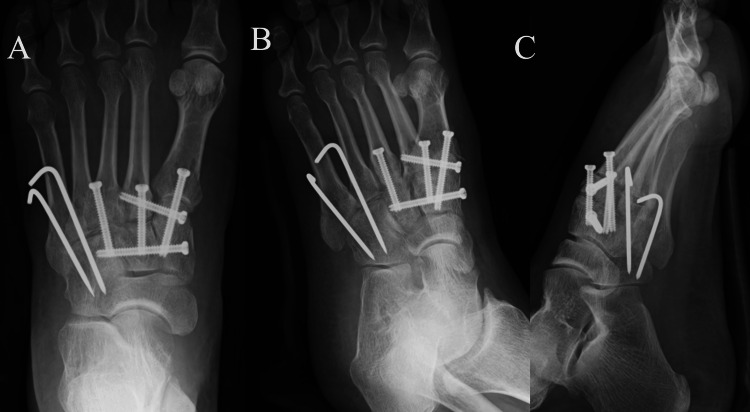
Postoperative radiographs demonstrating definitive fixation (A) Anteroposterior view. (B) Medial oblique view. (C) Lateral view. Cannulated cancellous screws and Kirschner wires (K-wires) were used to stabilize the Lisfranc joint and maintain anatomical alignment of the tarsometatarsal complex.
